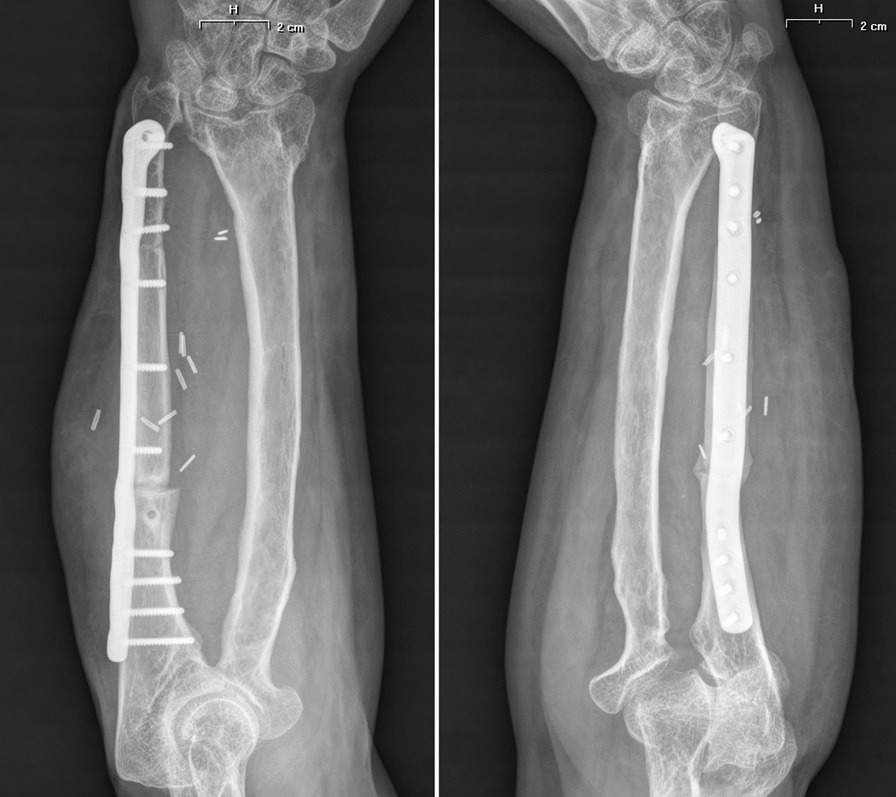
Follow-up X-rays at 8 months
